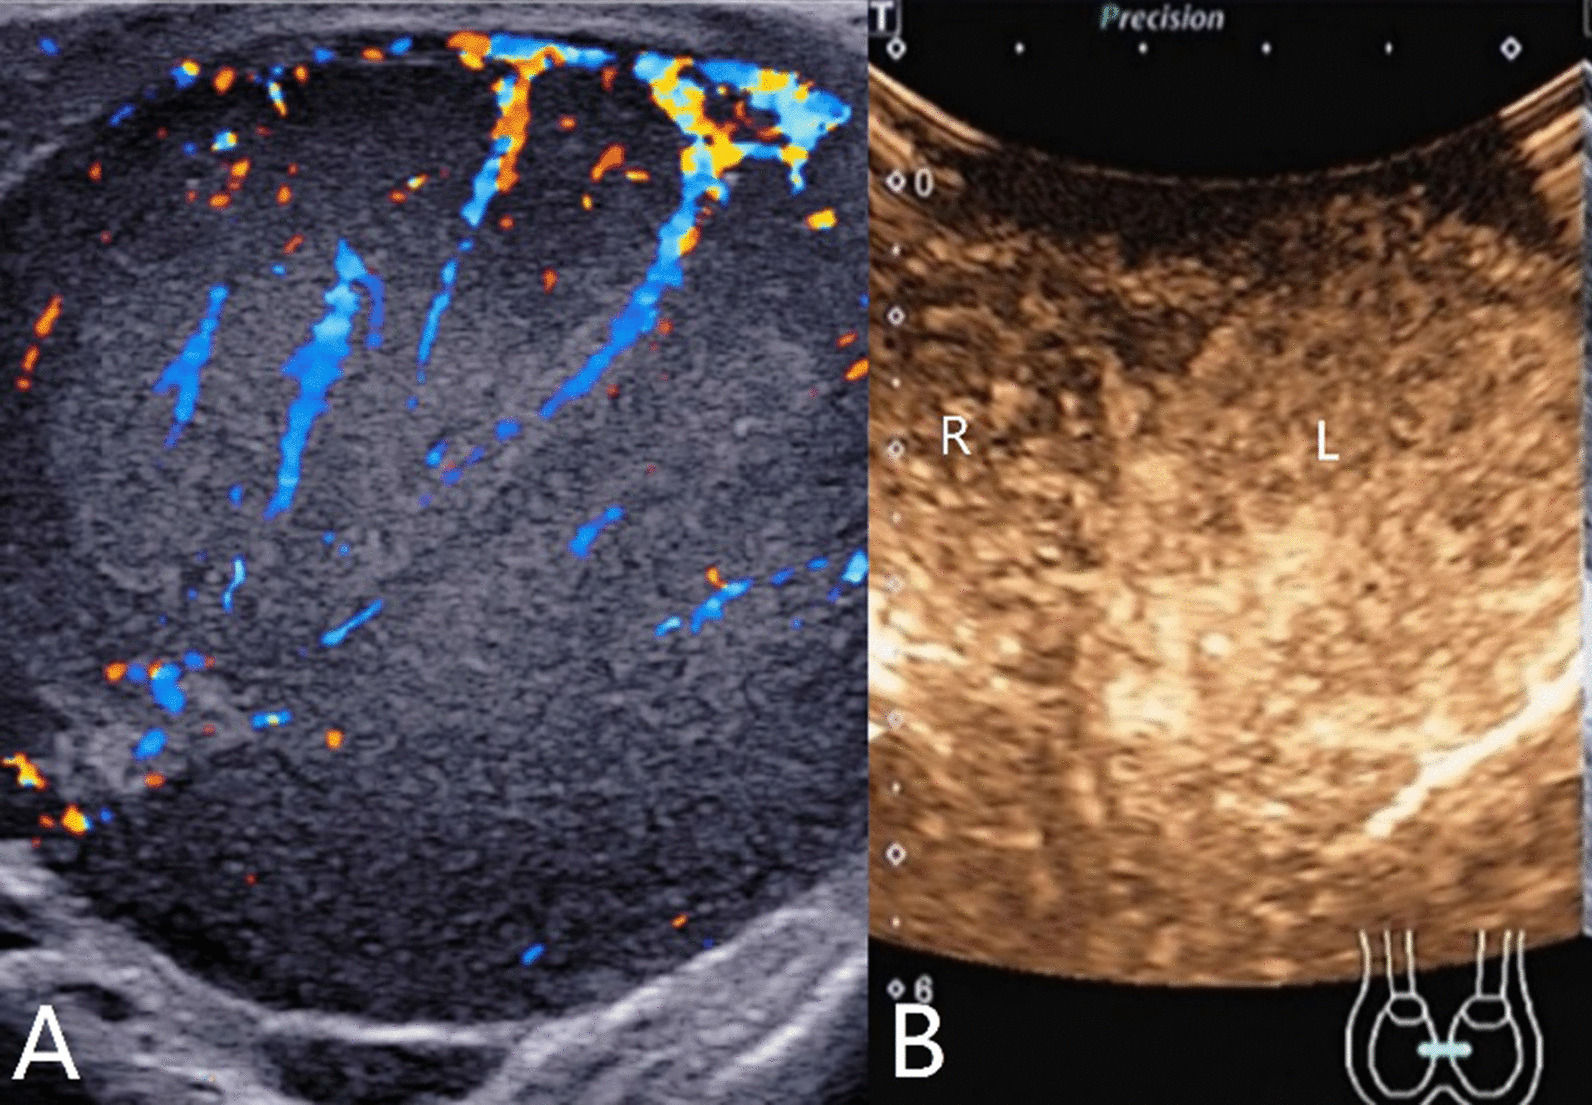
Testicular lymphoma. A Conventional ultrasonography showed that the left testis had a clear and hypoechoic border with abundant blood flow inside. B Contrast-enhanced ultrasonography shows uniform high enhancement. R: Right testis; L: left testis
